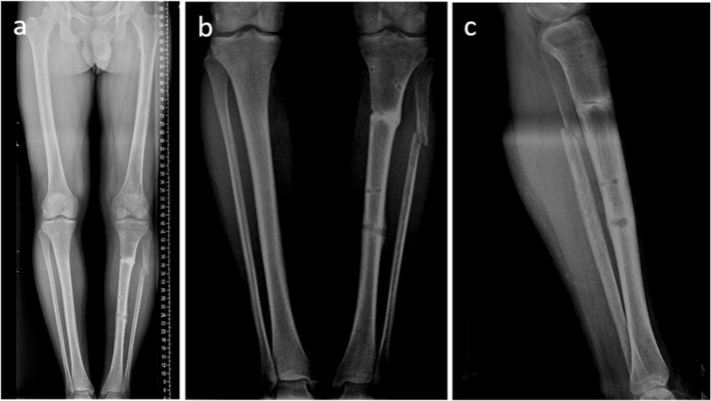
a–c The postoperative fifth-month follow-up radiographs of the patient showed complete union of the fracture
